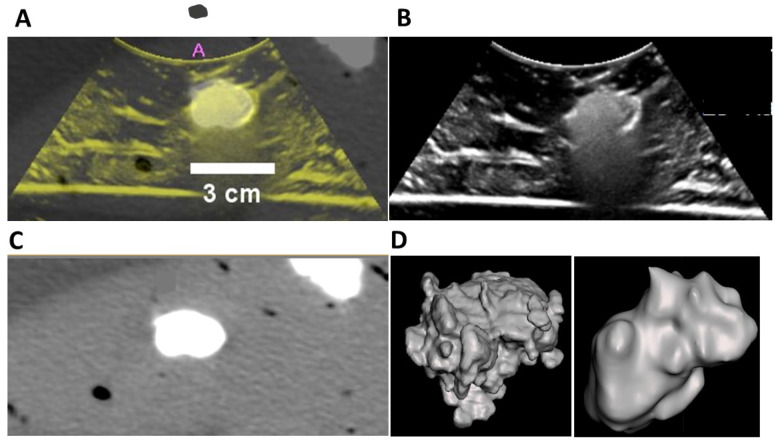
US and CT image fusion, and 3D distribution of POL from US and CT imaging. (A) US and CT co-registration from POL with 10 mg/mL (4 mL) injected with MSHN, and their individual US (B), and CT (C) images. (D) 3D distribution of POL gel obtained from US (left), and CT (right) imaging.
